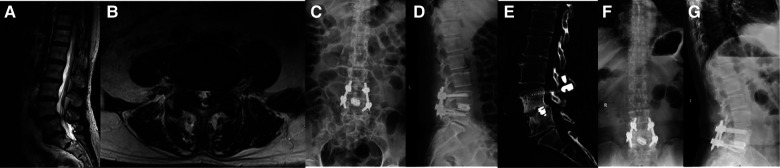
A 69-year-old female. Preoperative VAS-LBP: 6; Preoperative VAS-LP: 8; Preoperative ODI: 70%. (A, B) A L4/5 spinal stenosis identified in the preoperative MRI. (C, D) A good implantation position shown by x-rays taken a week after the operation. (E) At 12 months after the surgery, a CT scan image revealed a standard lumbar fusion. (F, G) The final x-ray images indicated that the implantation occurred in a good position.
